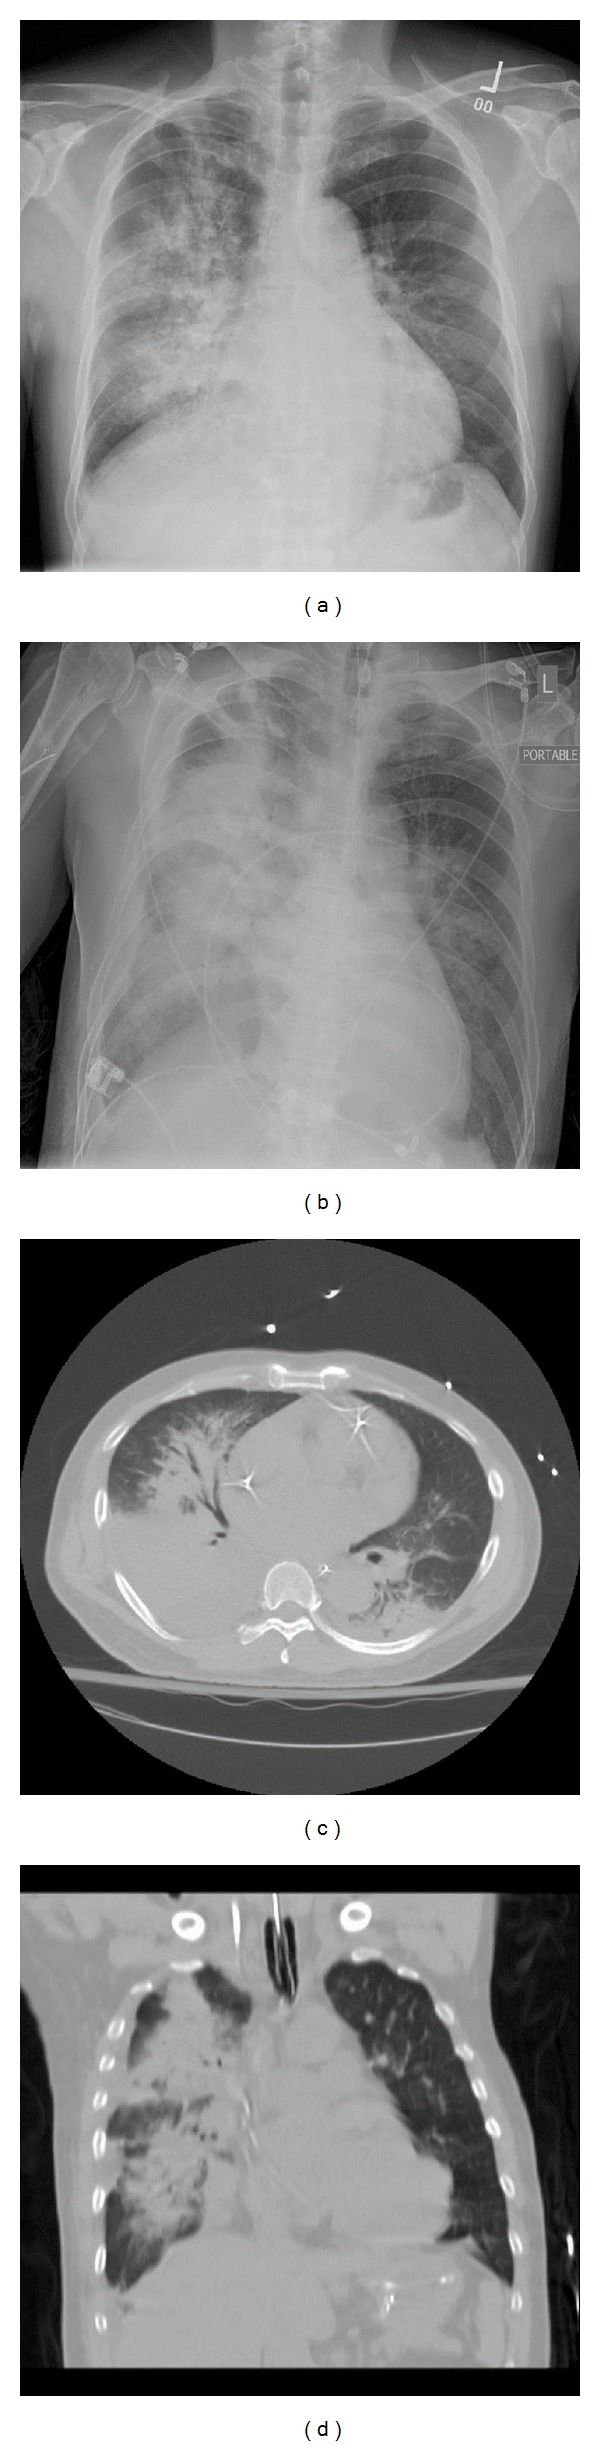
(a) CXR showing right sided fluffy infiltrates during the initial presentation. (b) CXR showing a worsening of infiltrates accompanied by pulmonary edema. (c) and (d) Sagittal and transverse chest CT sections confirming the presence of dense infiltrates predominantly located in the right upper and middle lobes.
